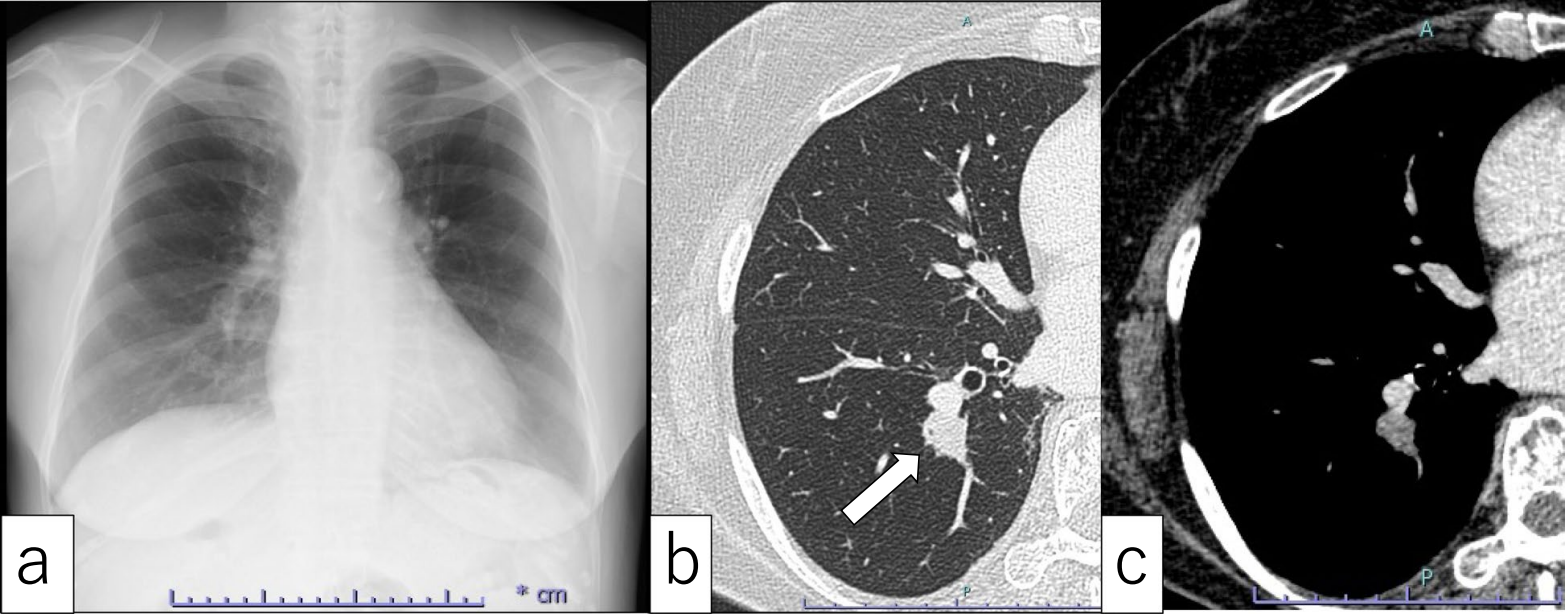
a Chest radiograph showing no obvious abnormal shadows. b Axial CT showed a nodule of 20 mm in diameter on the central side of S10 in the right lower lobe of the lung (arrow). c Axial contrast CT of the mediastinal window showed a relatively low density nodule with weakly heterogenous concentration
